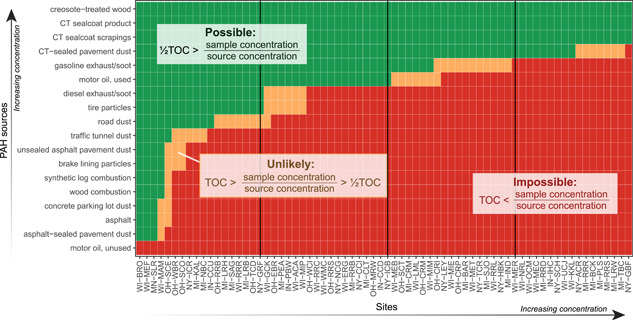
The likelihood of different polycyclic aromatic hydrocarbon (PAH) sources to be the primary source to individual streambed sediment samples, based on PAH concentration and total organic carbon (TOC) in samples versus PAH concentration in sources. Source concentrations are means of up to 12 samples compiled from previous studies (Supplemental Data, Table 3). Sites are ordered by TOC‐normalized PAH concentration. CT = coal tar. For site abbreviations, see Table 1.
